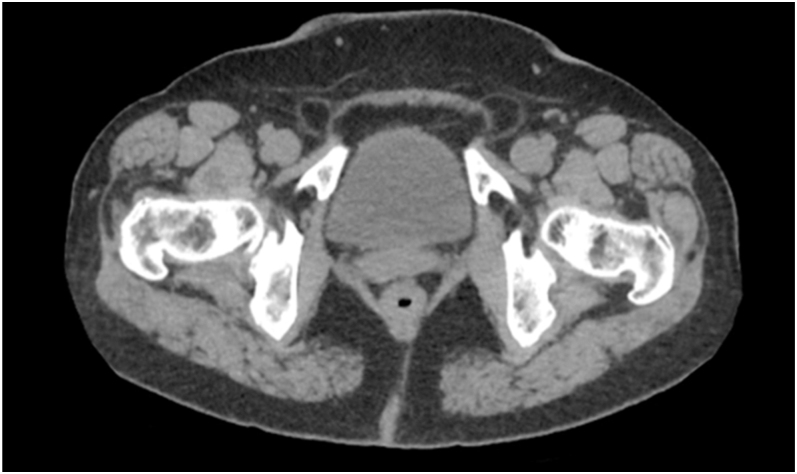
Abdominal CT almost 2 years after parathyroidectomy, without intestinal abnormalities.
